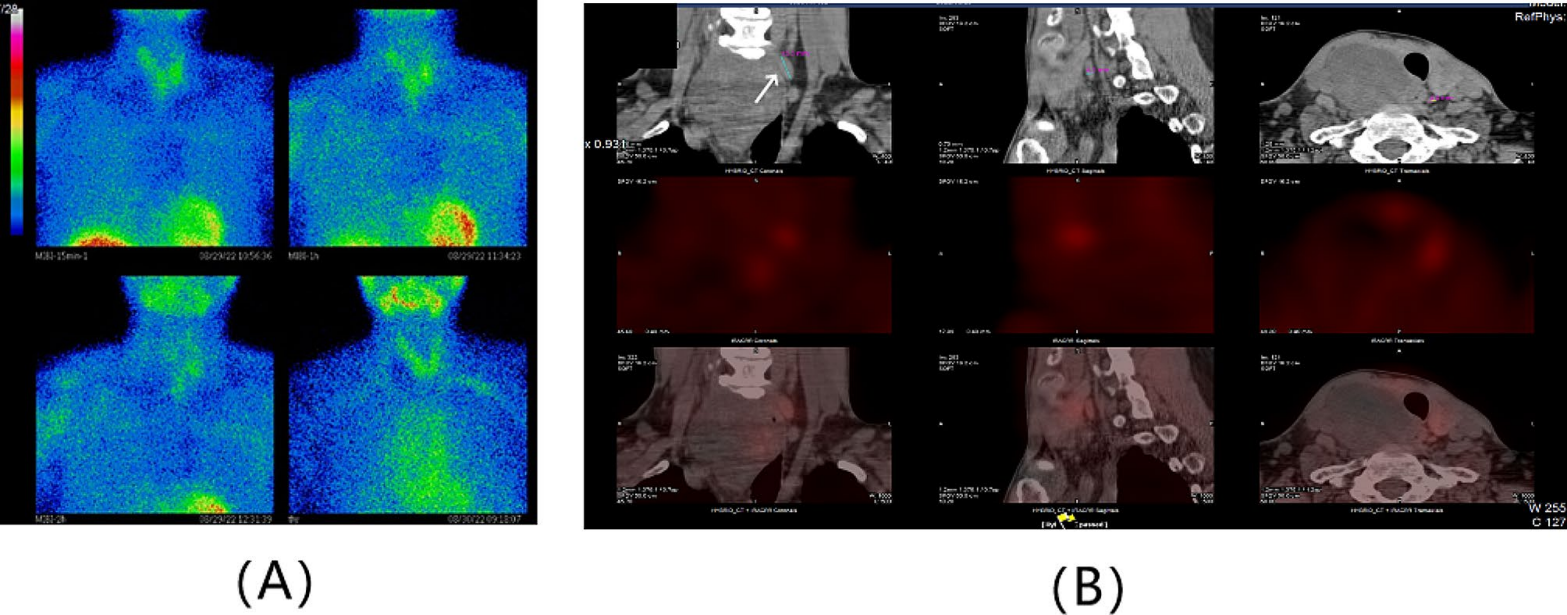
Slight uptake signal at the posterior tubercle of the superior left thyroid lobe in 99Tc-sestamibi dual-phase SPECT (A) and parathyroid fusion imaging showed parathyroid adenoma in 15.5 mm*4.3 mm*4.8 mm (B)
